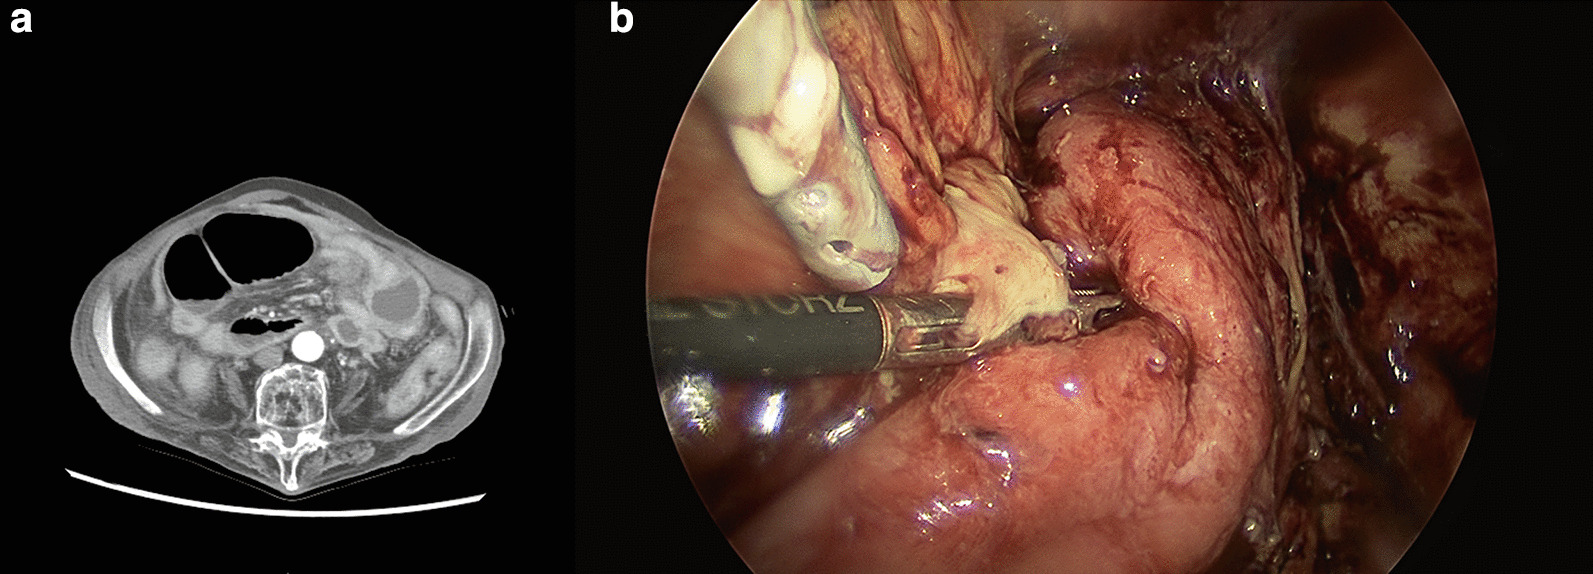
a CT scan of the patient. b A small intraabdominal abscess was found during surgery
